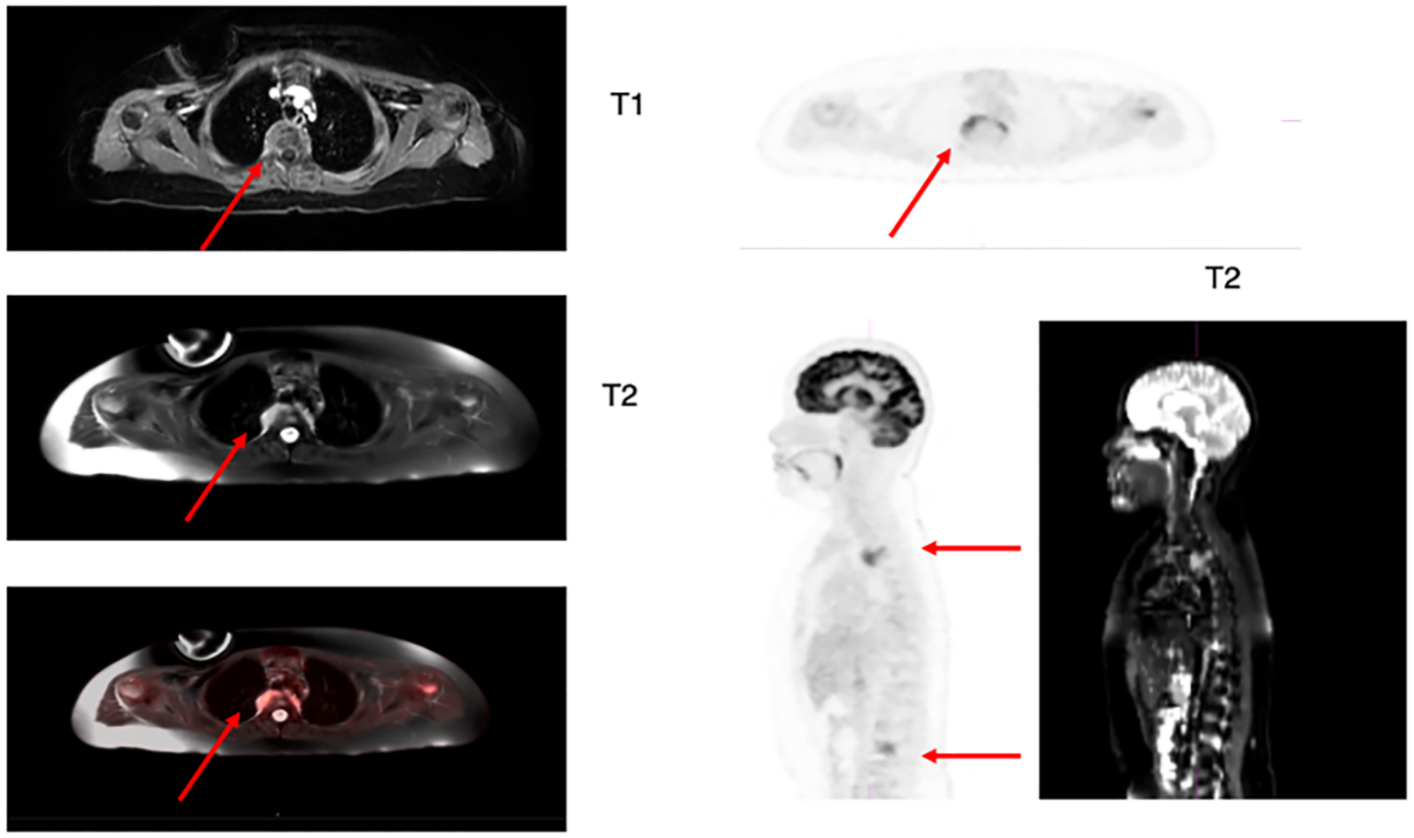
Axial and sagittal 18-FDG PET/MRI T1 and T2 weighted images showing hypermetabolic focal uptake in T2-T4 level and L3-L4 level. The MRI shows abnormal increased signal on axial view of the right posterior side of thoracic vertebral level with corresponding increased FDG activity on the PET scan images (arrow). The lumbar lesion is best identified on the sagittal views as increased metabolic activity in the lumbar spine on 18F-FDG PET and increased signal more focally in the spine in the areas of focal increased FDG activity (arrows).
